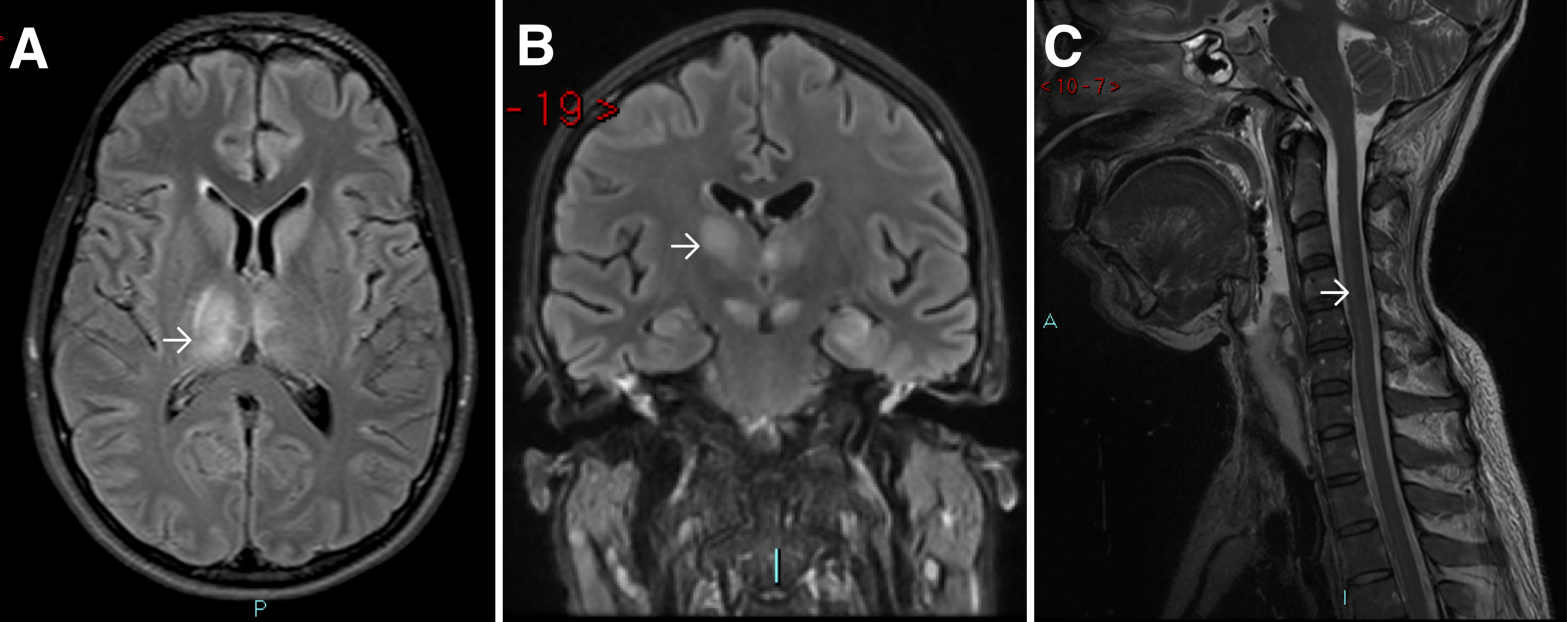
Neurological imaging of Patient two. (A), MRI scan performed in Bangkok on 18 July 2015 showing bilateral thalamic lesions, worse on the right (arrow). (B and C), MRI scan in Liverpool on 4 August 2015 showing persistent thalamic lesions (B, arrow) and high signal in the spinal cord (C, arrow)
